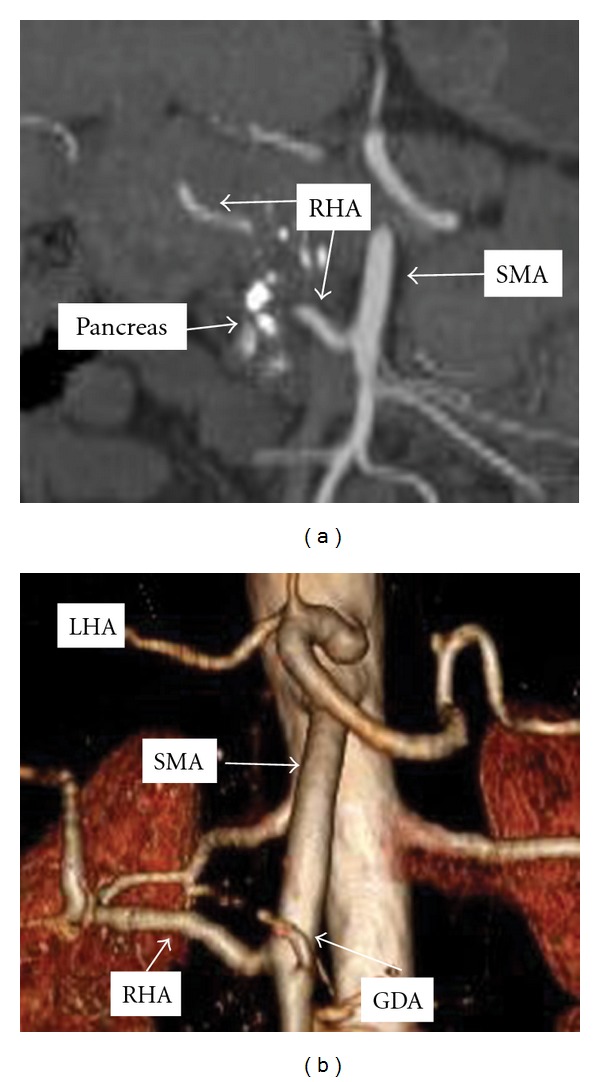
Coronal computed tomography reconstruction of mesenteric arterial vasculature in Case 2. GDA: gastroduodenal artery, LHA: left hepatic artery, RHA: right hepatic artery, SMA: superior mesenteric artery, Pancreas: calcified head of the pancreas.
